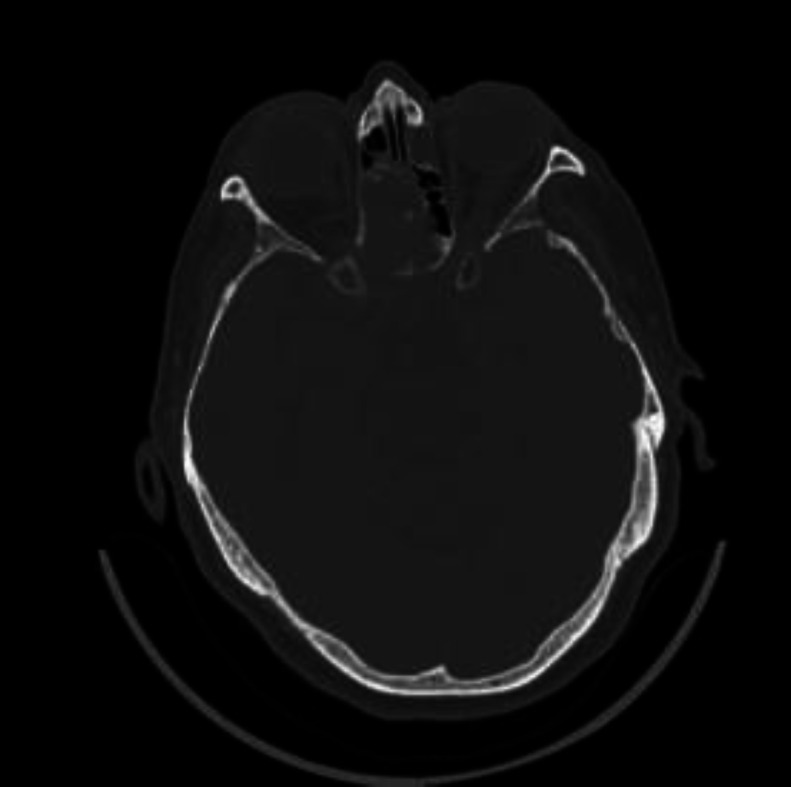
Meningoencephalocele post-op CT
